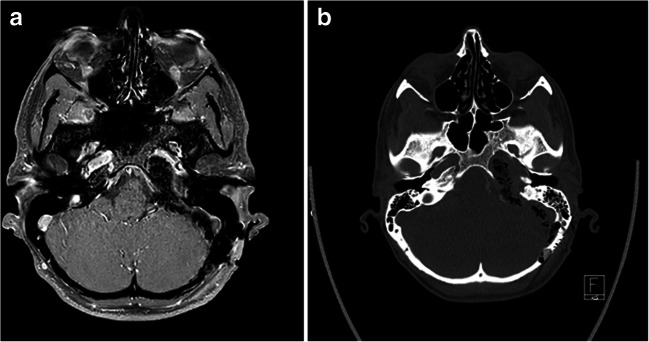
Postoperative imaging demonstrating near-total resection of the chondrosarcoma in the petrous apex left side on axial T1 Gd+ MRI subtraction series (a) and CT (b)
